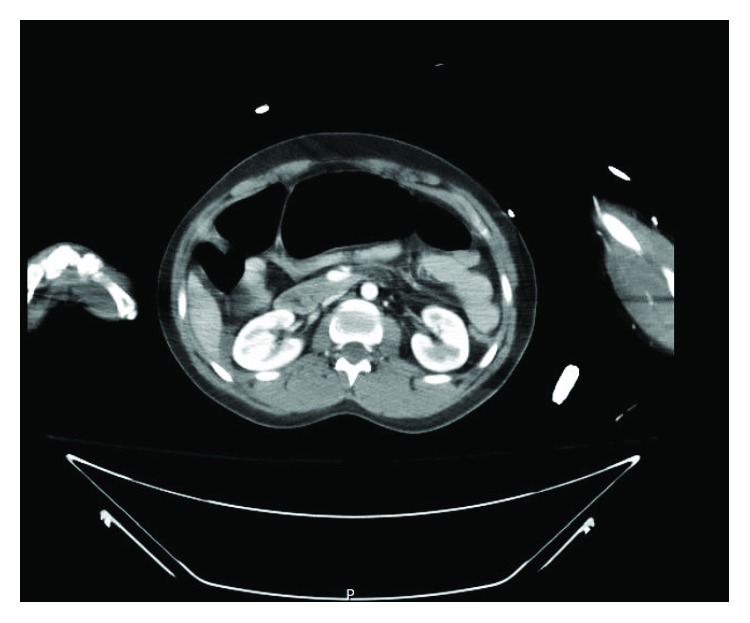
This is the CT scan obtained on the day of admission following the motor vehicle crash. Note the absence of any radiographic suggestion of duodenal obstruction by the SMA. Also note the relatively small size of the IVC as a reflection of volume depletion on presentation and the free blood in the right gutter.
